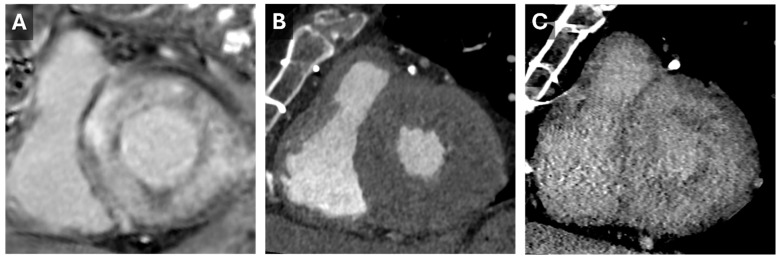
Cardiac Amyloid Findings. CMR short-axis delayed-enhanced inversion recovery image through the LV base (A) from a patient with severe cardiac amyloidosis with diffuse transmural delayed enhancement. Short axis images through the LV base from a previous cardiac CT with arterial (B) and 5 min delayed (C) phases. The delayed phase demonstrates diffuse nonvascular enhancement that correlates well with the findings seen on the subsequent CMR.
